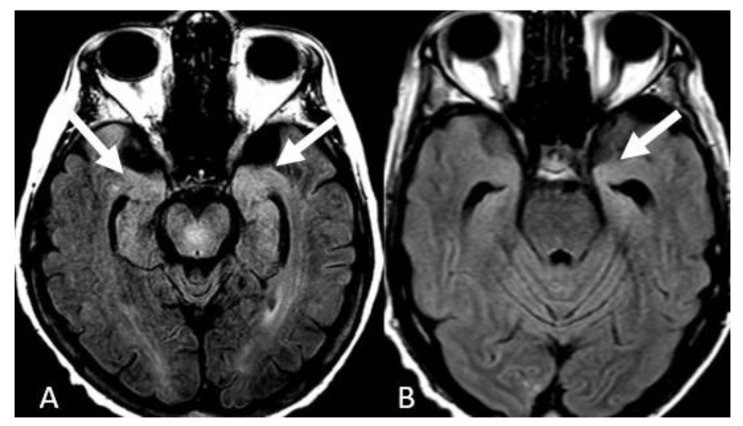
Temporal lobe involvement. (A) Axial FLAIR images depict bilateral medial temporal lobe FLAIR hyperintensities. (B) Isolated left medial temporal FLAIR hyperintensities. Image has been windowed manually to improve the visibility of signal abnormality.
